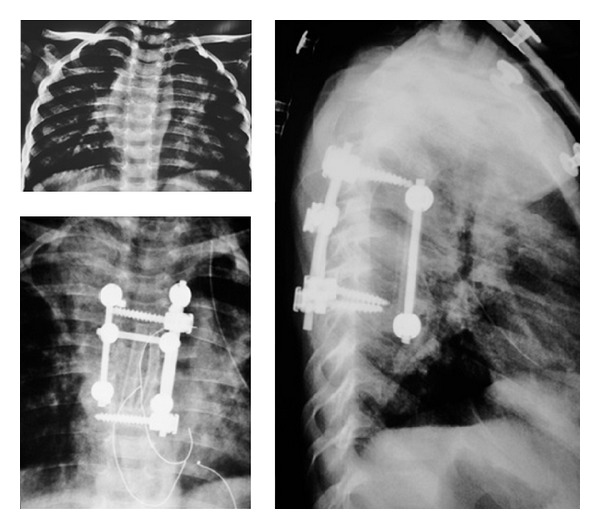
Preoperative and postoperative plain roentgenograms.
